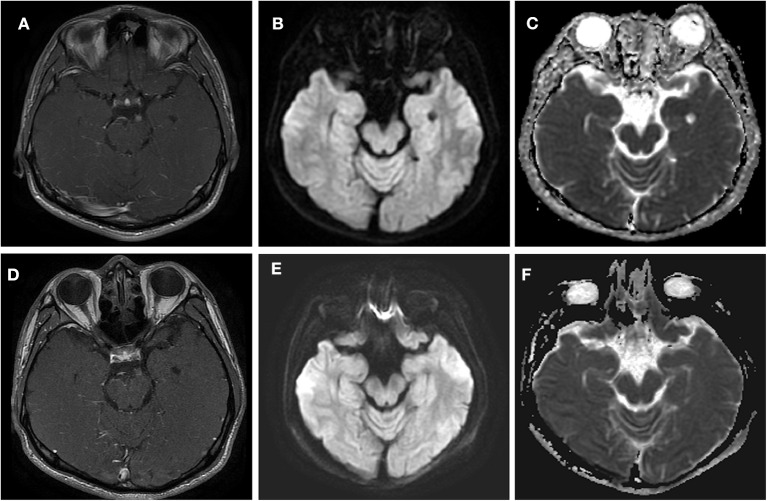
Axial T1 contrast-enhanced MRI (44 days after headache) and DWI (30 days after headache) through the point at which the oculomotor nerve emerges from the midbrain in this patient with left oculomotor ophthalmoplegic migraine. (A–C) Acute phase. The MRI shows a nodular, enhancing lesion in the cisternal segment of the left third nerve (arrow) in (A). In (B,C), a signal was demonstrated at the midbrain of the left-sided third cranial nerve on DWI and ADC. (D–F) Quiescent phase, taken 7 weeks after the left ptosis and diplopia had resolved. (D) Demonstrates the decreased enhancement. (E,F) Demonstrate that the signal had disappeared at this point on DWI and ADC.
